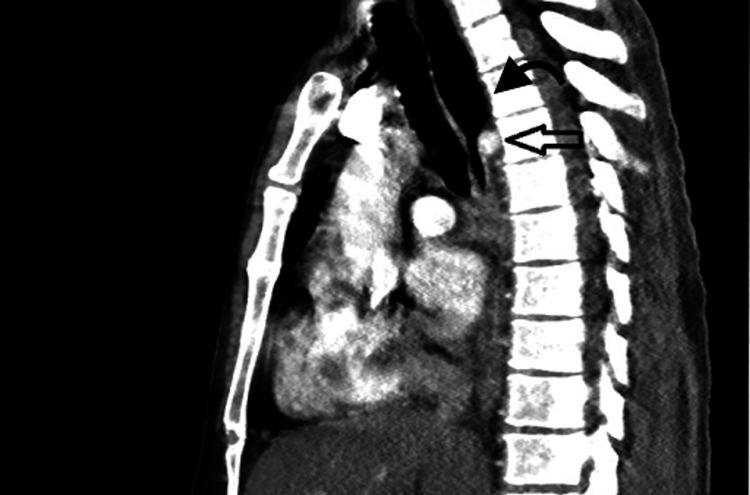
Chest CT angiography Chest CT angiography shows aberrant right subclavian artery (arrow), causing a mild mass effect on the upper esophagus and dilated patulous esophagus (curved black arrow) containing fluid with diffuse circumferential wall thickening in the mid and distal aspects.
